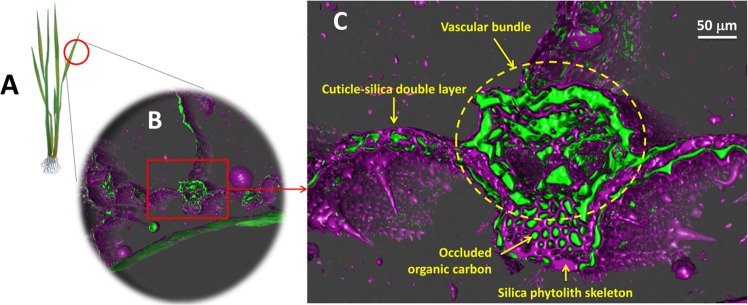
Occlusion of organic carbon in rice straw phytoliths: (A) rice plant, (B) X-ray tomographic image showing the rice leaf, and (C) a part of the leaf containing the silica phytolith skeleton (coloured in violet) and subcompartmentated organic matter (coloured in green).
